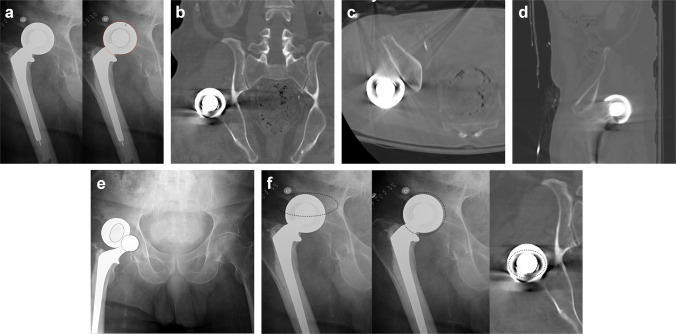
a Anterior/posterior radiograph of the pelvis demonstrating dislocation of right hemiarthroplasty prior to any attempts at reduction. Left: unannotated image. Right: annotated overlay highlighting the “O”-shaped borders of the outer bipolar shell (red ring) and inner femoral head (black ring). Arrow denotes asymmetric radiodensity from anterior rotation of the outer shell. b Coronal CT with contrast shows the outer shell of the bipolar head anteriorly rotated. A concentric O-shaped radiodense rim with asymmetric wall thickness is seen, with the eccentrically positioned femoral head producing an elliptical cavity configuration. c Axial CT with contrast confirms anterior rotation of the outer shell of the bipolar head with asymmetric wall thickness. Eccentric positioning of the femoral head results in uneven spacing and a distorted central cavity configuration. d Sagittal CT with contrast demonstrates a relatively uniform appearance of the outer shell, with decreased evidence of anterior rotation. However, the femoral head remains eccentrically positioned within the outer shell, evidenced by the asymmetric spacing between components. e Anterior/posterior radiograph showing bipolar head dissociation following closed reduction. Dashed-ring annotations highlight separation of the outer acetabular shell from the inner femoral head of the component. f Radiographic and CT indicators of bipolar cup dissociation risk. Anteroposterior radiograph shows an apparently concentric, radiodense “O” outline of the outer shell; however, subtle asymmetric cortical thickness of the ring suggests anterior rotation. Coronal CT confirms eccentric seating of the inner femoral head within the shell, indicating misalignment despite the deceptive concentric silhouette, a pattern associated with failed or hazardous closed reduction attempts and potential intrareduction dissociation
